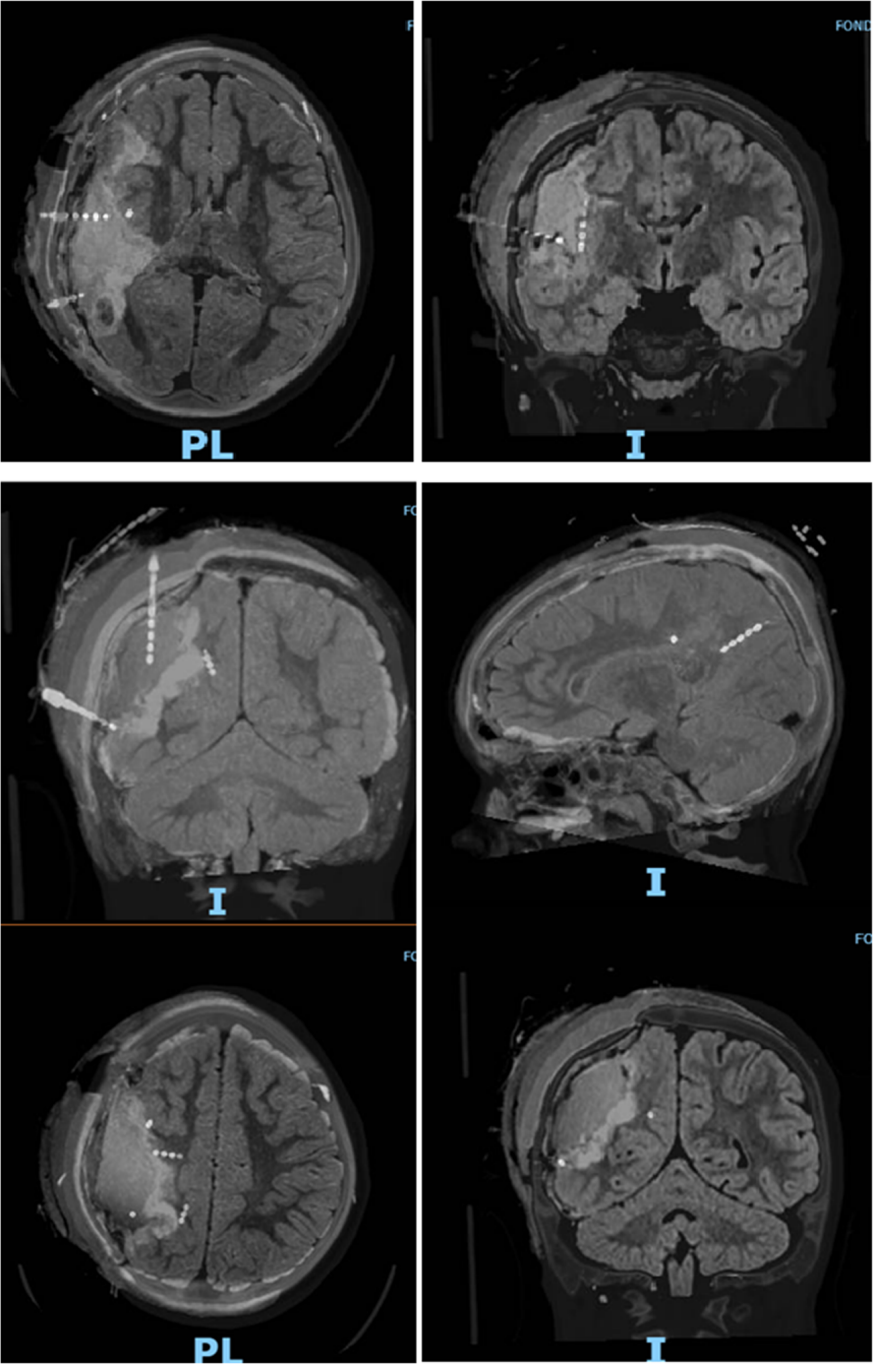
Post-surgical MRI with superimposition of CT sequences for SEEG electrodes localization confirming the removal of the identified epileptogenic areas
